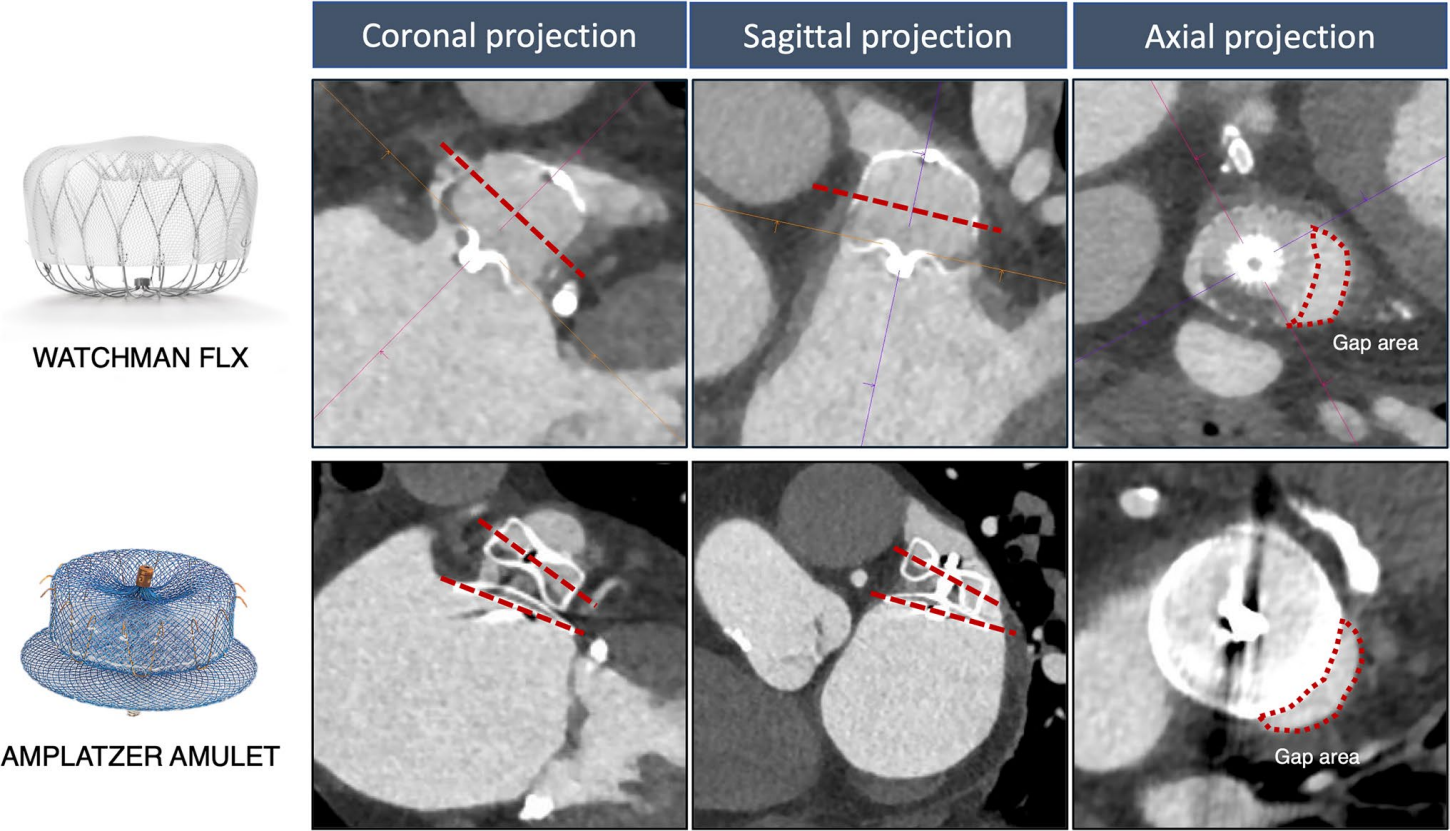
Cardiac CT multiplanar reconstructed views for investigation of peridevice leak. The dotted lines represent the proximal and mid-device cross-sectional views (Watchman FLX device, top panel) and disc and mid-lobe cross-sectional views (Amplatzer Amulet device, bottom panel)
